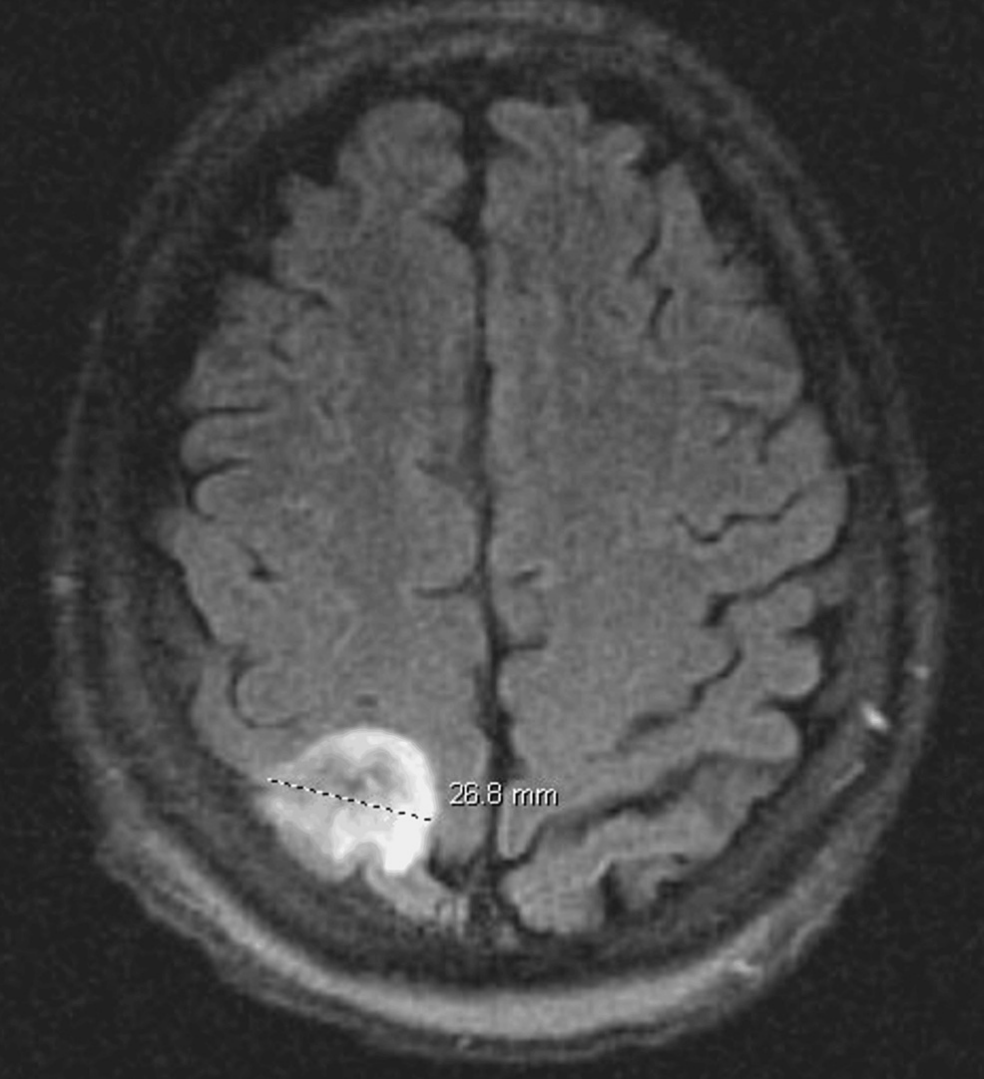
T2/FLAIR hyperintense lesion in R postcentral gyrus with minimal central restricted diffusion and mild associated surrounding vasogenic edema FLAIR: fluid-attenuated inversion recovery
